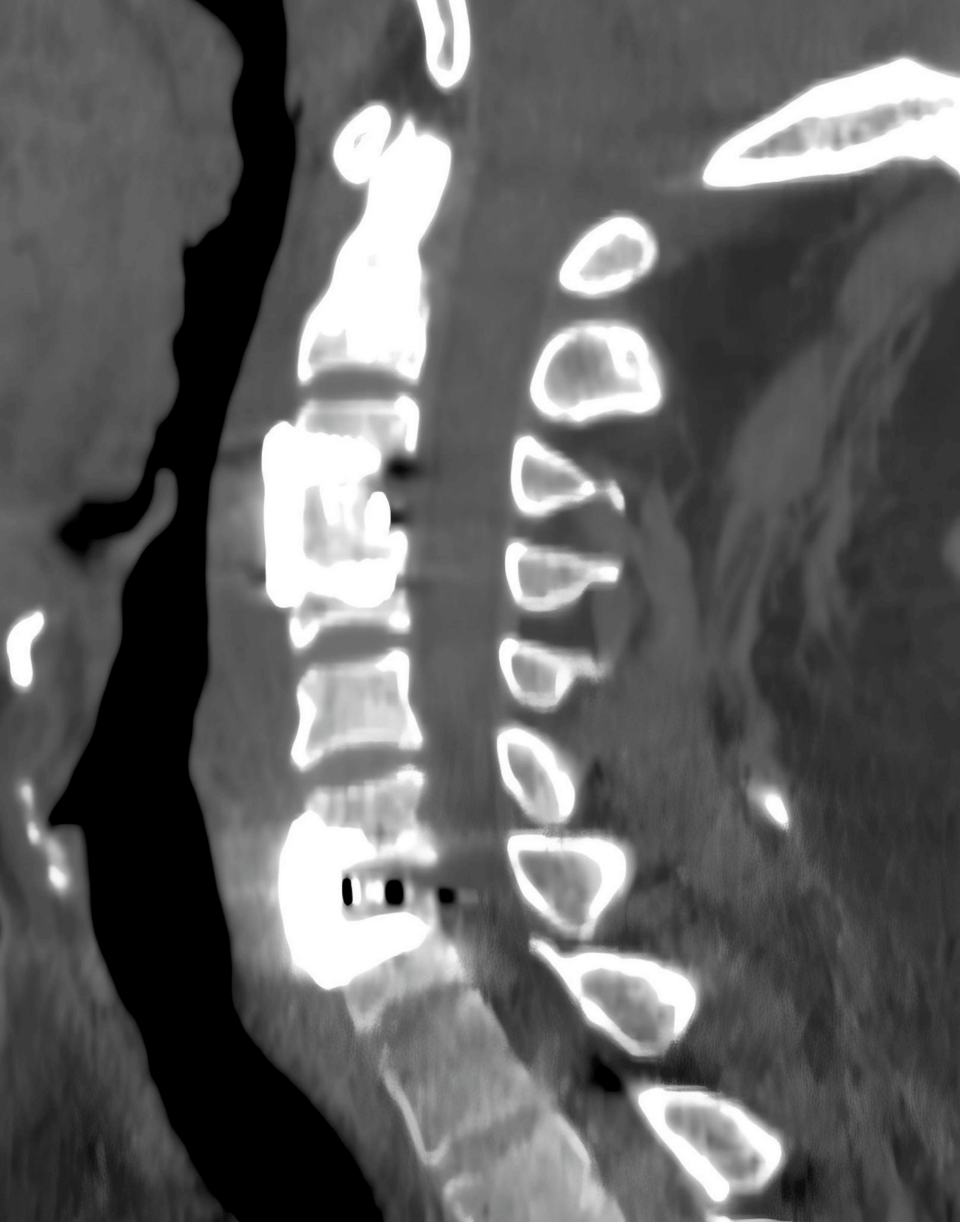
CT scan one month after surgery One month after the surgery, CT scan showed that the cervical hematoma was completely resected and the internal fixation was in a good position.
